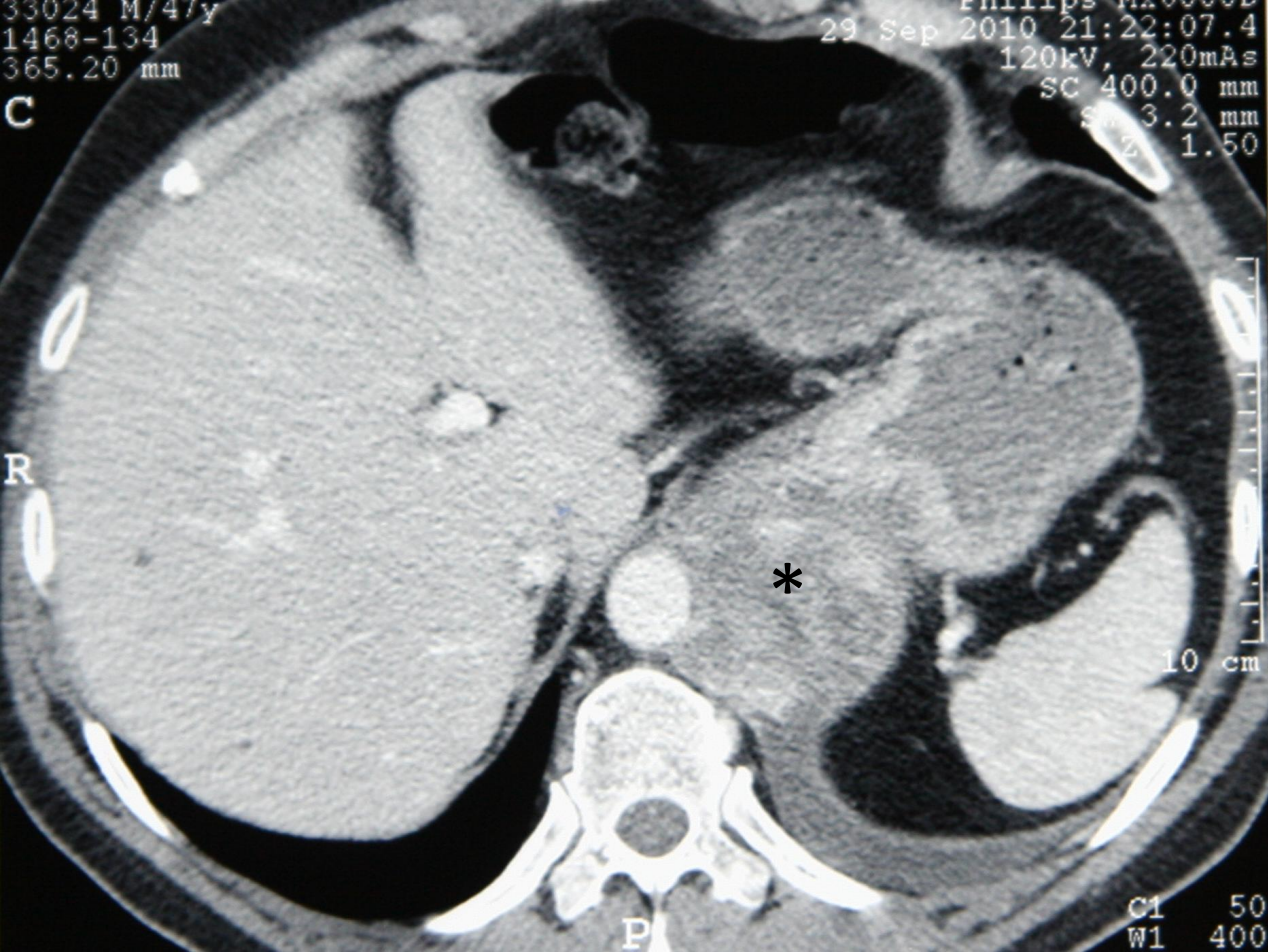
Preoperative contrast-enhanced CT revealing the thoracoabdominal sarcoma mimicking aortic rupture. Notice the enhancement outside the aorta (asterisk).
